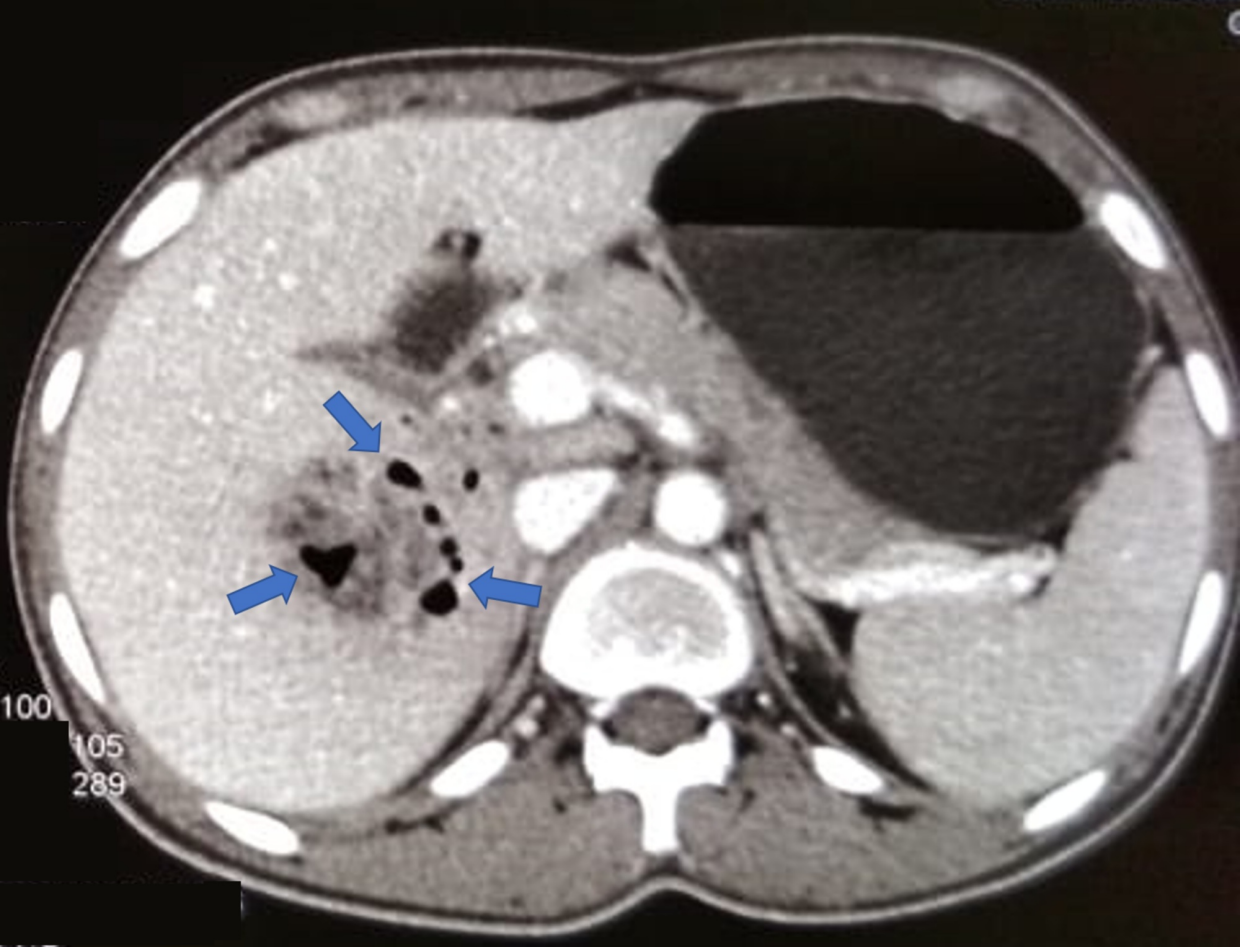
Contrast-enhanced computed tomography of the abdomen, showing liver abscesses
